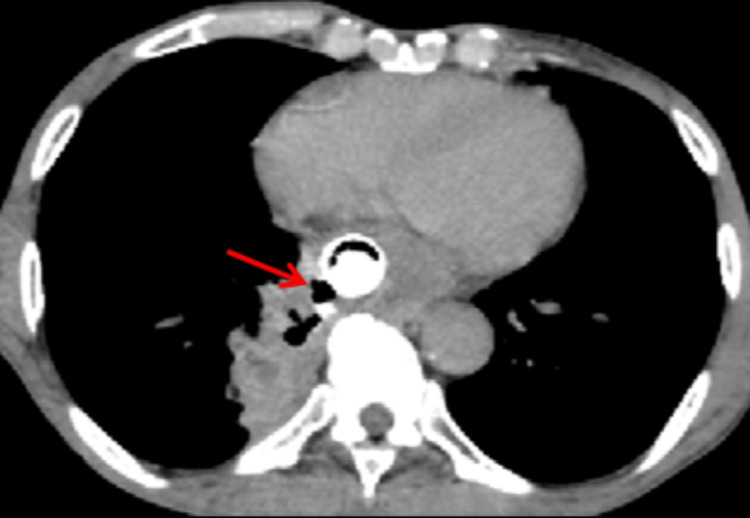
CT contrast phase - axial section showing contrast leak and luminal wall thickening
